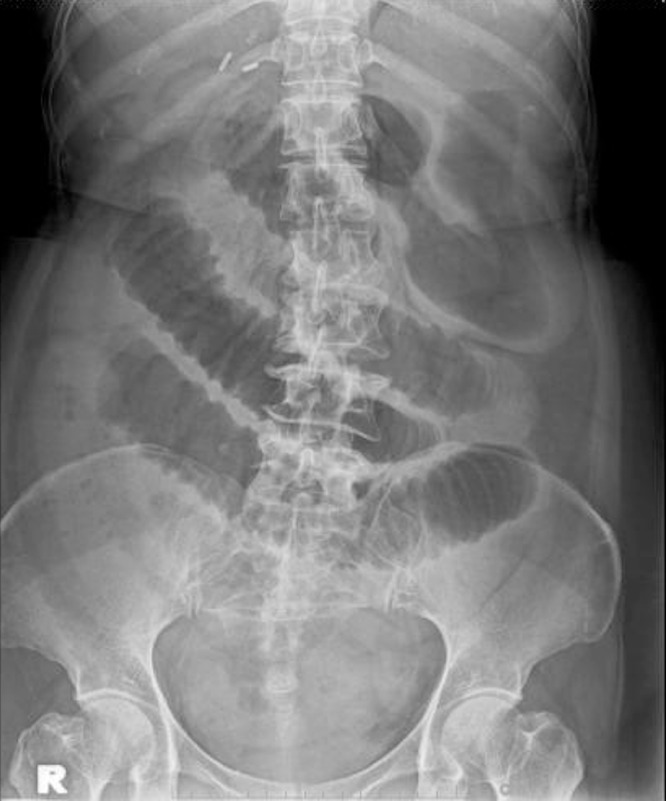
Abdominal radiograph showed a dilated small bowel with no air in the large bowel.
